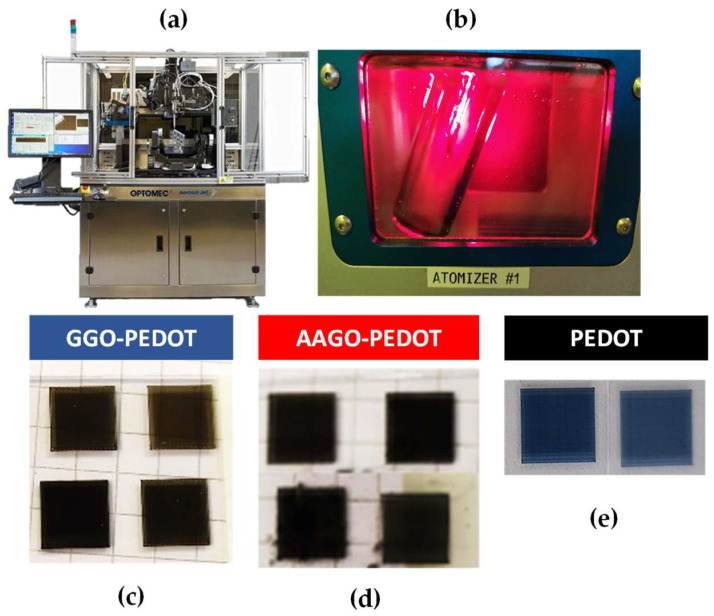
(a) Aerosol Jet^®^ Printing machine commercialised by Optomec Inc. (Albuquerque, NM, USA), (b) Atomiser in the ultrasonic configuration of Aerosol Jet^®^ Printing machine, (c) GGO-PEDOT thin films printed on a glass substrate, (d) AAGO-PEDOT thin films printed on a glass substrate, (e) PEDOT thin films printed on a glass substrate.
